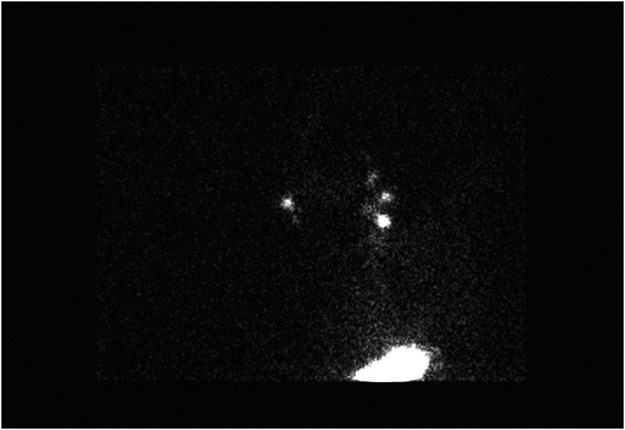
Lymphoscintigraphy showing four inguinal sentinel nodes (three ipsilateral and one contralateral).
